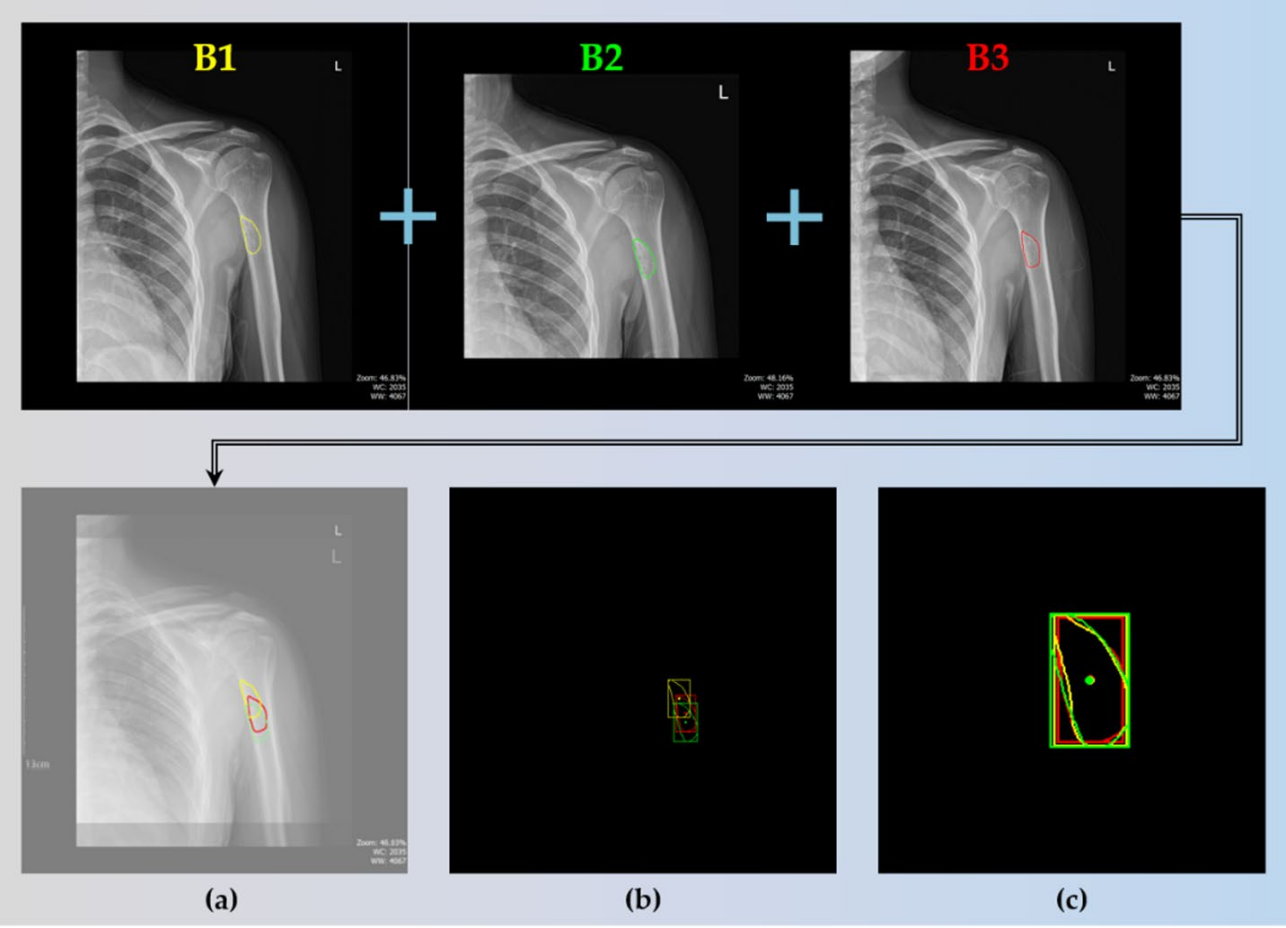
X-ray images B1, B2, and B3 acquired at one-year intervals. (a) Overlaid image combining B1, B2, and B3 to visualize changes over time. (b) Cropped edges of the BBT regions with corresponding bounding boxes and computed centroids. (c) BBT regions realigned based on their centroids to assess shape variations across time points.
